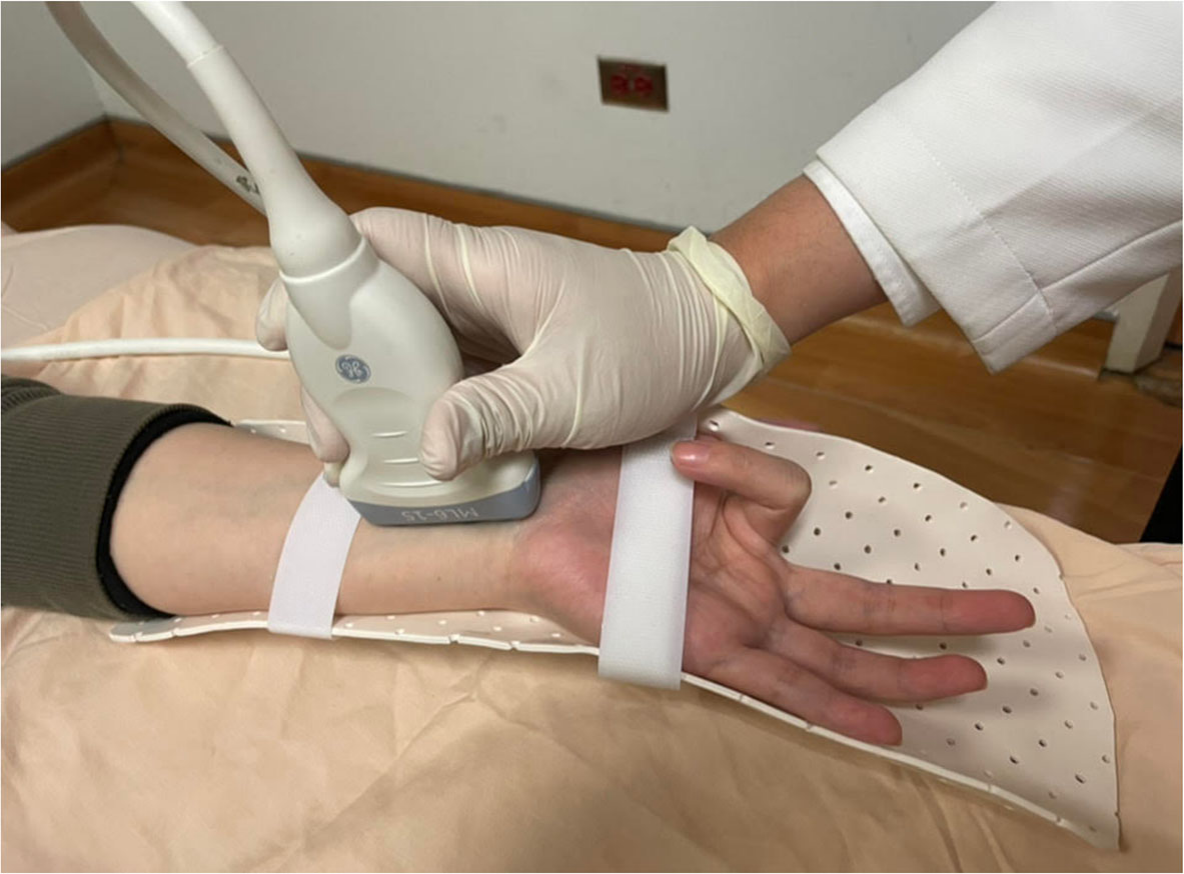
Study setup with the examinee’s hand on a splint with 30° extension and the ultrasound transducer longitudinally placed on the wrist
